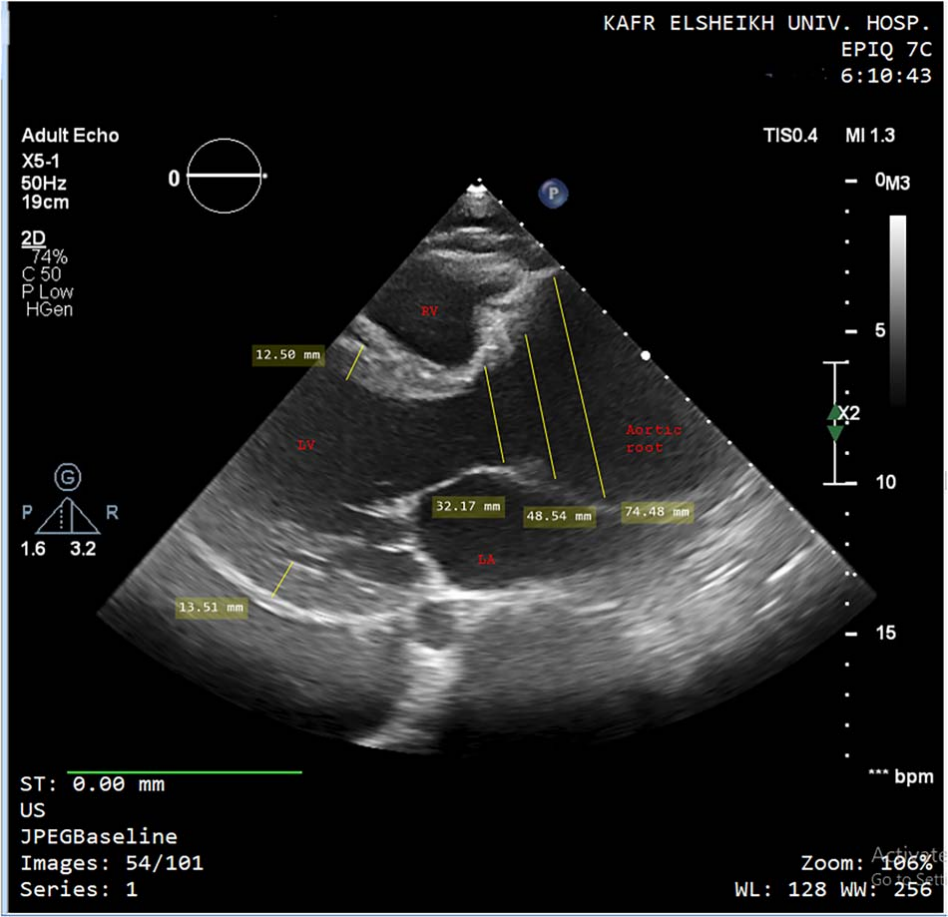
Transthoracic echocardiography showing the LV hypertrophy and the aortic root dilatation.
